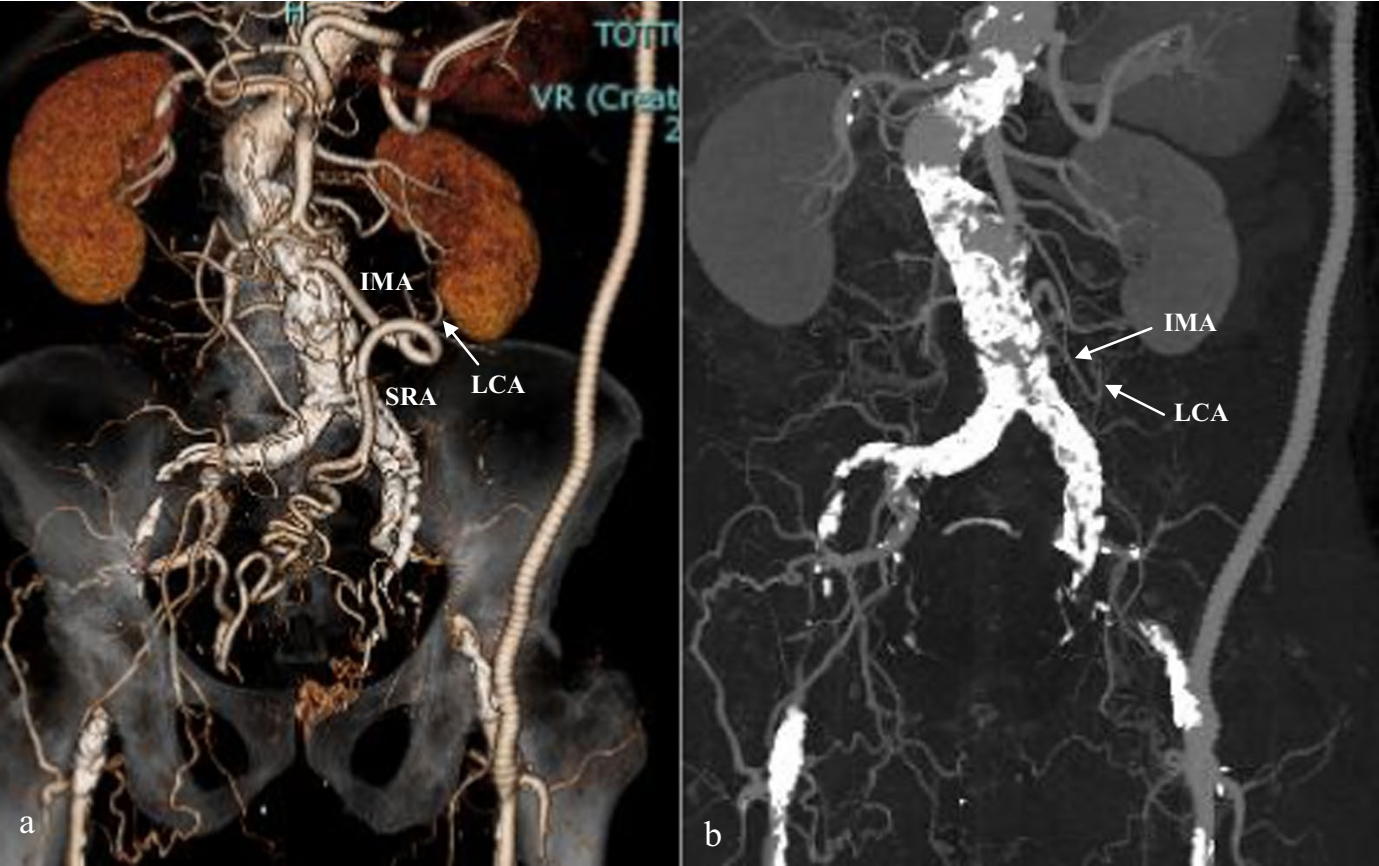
Computed tomography angiography taken after the surgeries. a After the axillofemoral bypass. Excellent blood flow to the left extremity through the bypass was proven. b After the laparoscopic sigmoid colectomy with inferior mesenteric artery preservation, composed of preservation of the inferior mesenteric artery to left colic artery and ligation of the superior rectal artery
